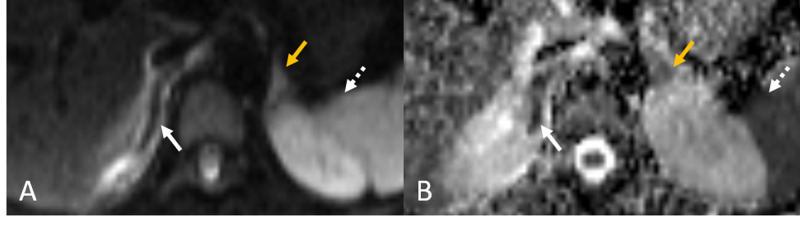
DWI images (A) with corresponding ADC map (B). In Figure A, the left adrenal gland (golden arrow) and spleen (white dashed arrow) show normal bright, hyperintense signal on DWI sequence. To contrast, the infarcted adrenal gland is iso- to hypointense (white arrow). Corresponding restricted diffusion is seen in Figure B, indicated by hypointensity. Restricted diffusion as expected is seen in the left adrenal gland (golden arrow) and spleen (white dashed arrow). Of note, the right adrenal gland (white arrow) restricts diffusion more than the left or the spleen, indicated by its relative hypointensity when compared to the spleen and left adrenal gland on this ADC map. DWI: diffusion weighted imaging, ADC: apparent diffusion coefficient
